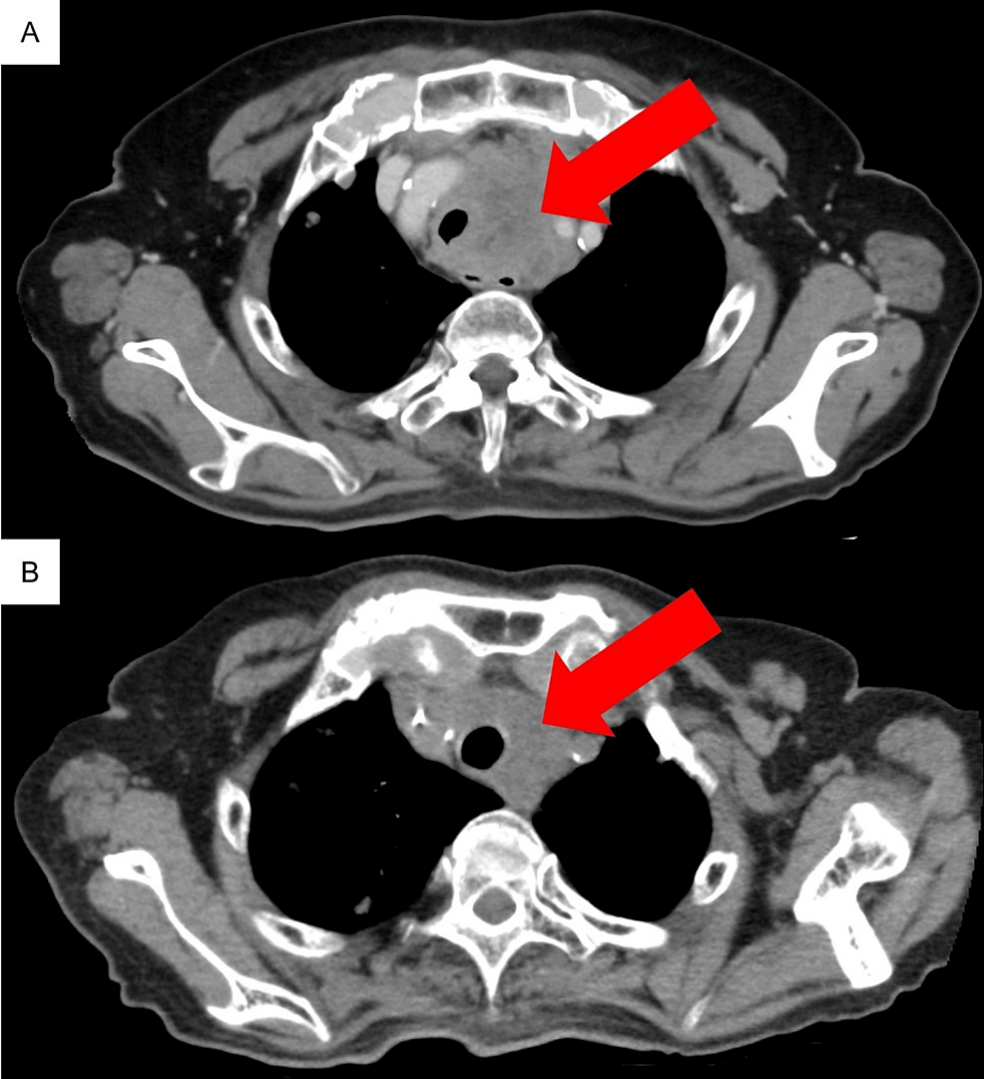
Cervical-thoracic computed tomography (CT) scans of the patient before and after radiotherapy. A) Preradiotherapy contrast-enhanced computed tomography (CT) images; B) postradiotherapy plain CT images. (A) Prior to radiotherapy, the trachea was compressed by the tumor and displaced to the right side (arrow). (B) Taken two weeks after radiotherapy, radiotherapy resulted in a marked shrinkage of the tumor and improvement in airway displacement (arrow).
